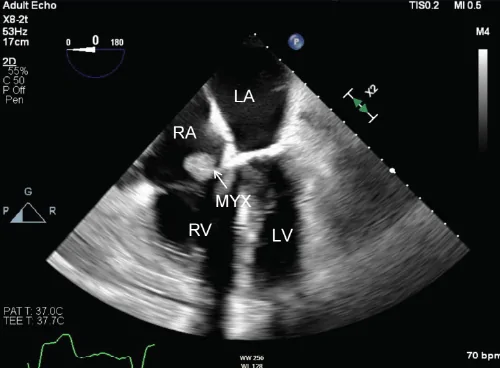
Transesophageal echocardiogram, mid-esophageal-chamber view. *RA*, right atrium; *RV*, right ventricle; *LA*, left atrium; *MYX*, myxoma.
